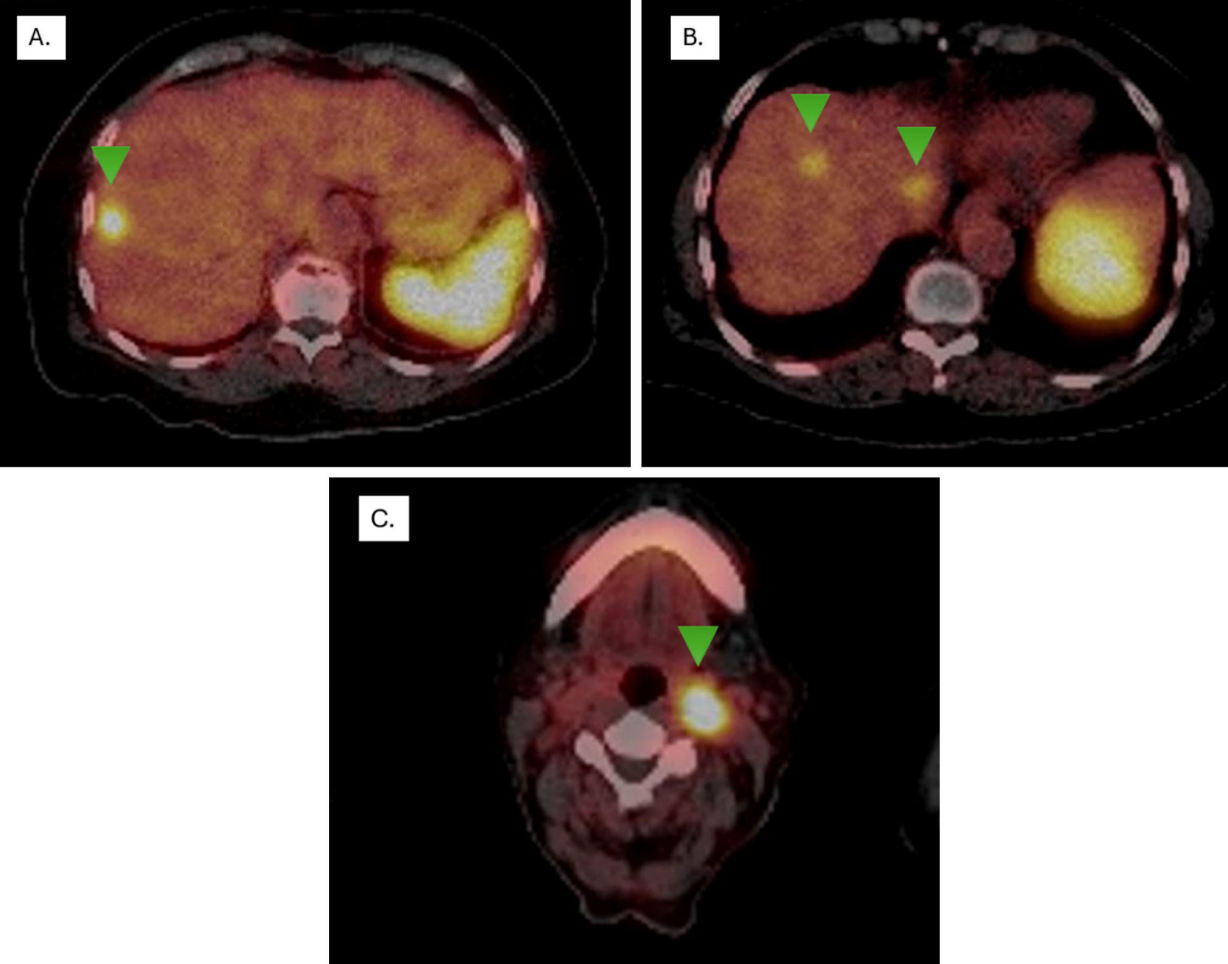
Ga-68 DOTA-péptido PETSCAN. Lesions with overexpression of somatostatin receptors (Green arrows) (A) segment VIII with a higher uptake, showing an SUVmax of 11.3 (previous study SUVmax 8.4), classified as Krenning 4, (B) segments VIII and I show SUVmax levels of 5.5 (previous study SUVmax 3.6) and 6.3 (previous study SUVmax 4.3), respectively, both classified as Krenning 3. (C) Lymph node in left station IIa, SUVmax of 40 (Krenning 4).
